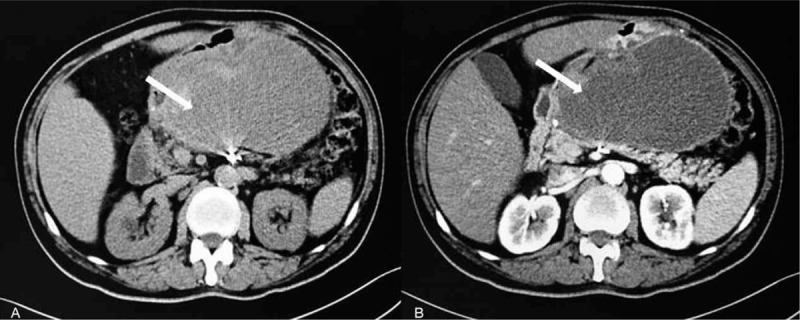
Enhanced CT scans 1 mo after intervention. (A) Non-contrast-enhanced phase. (B) Artery phase. White arrow shows the hematoma between the stomach and pancreas. CT = computed tomography.
